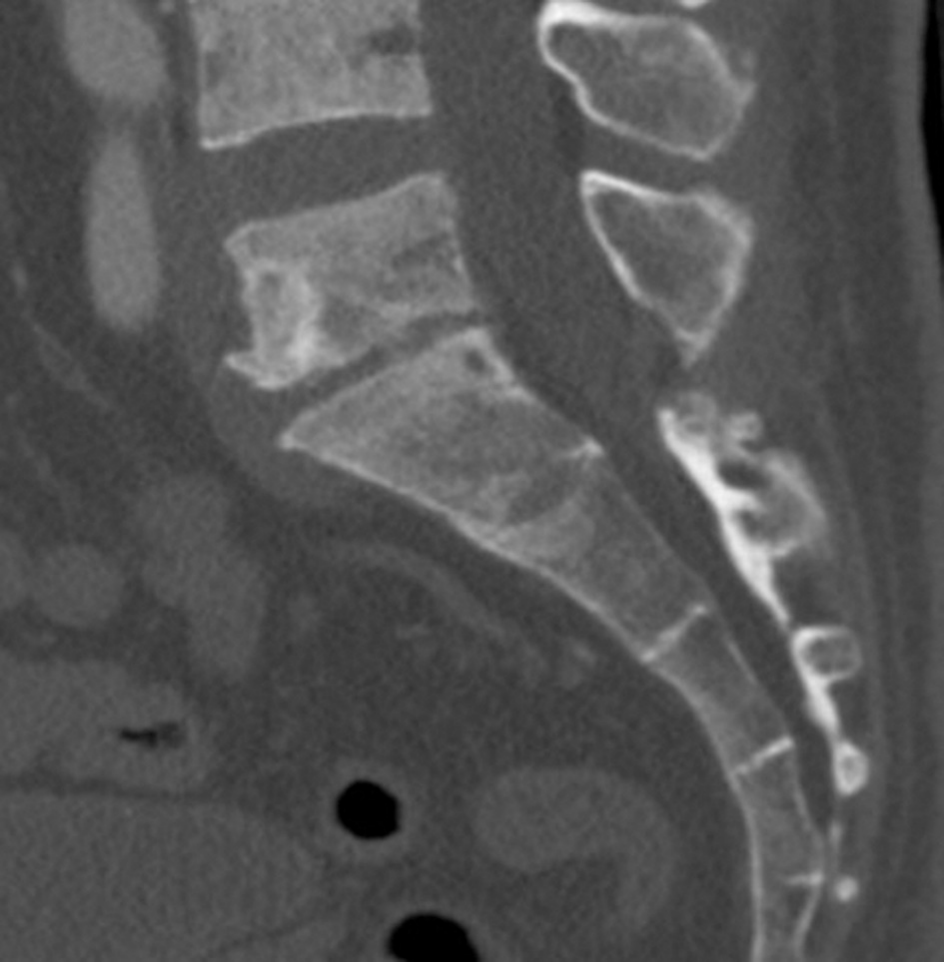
A sagittal CT image of the lumbar spine. The low-density tumour with a well-defined sclerotic margin involves the anterior aspect of the L5 vertebra.
